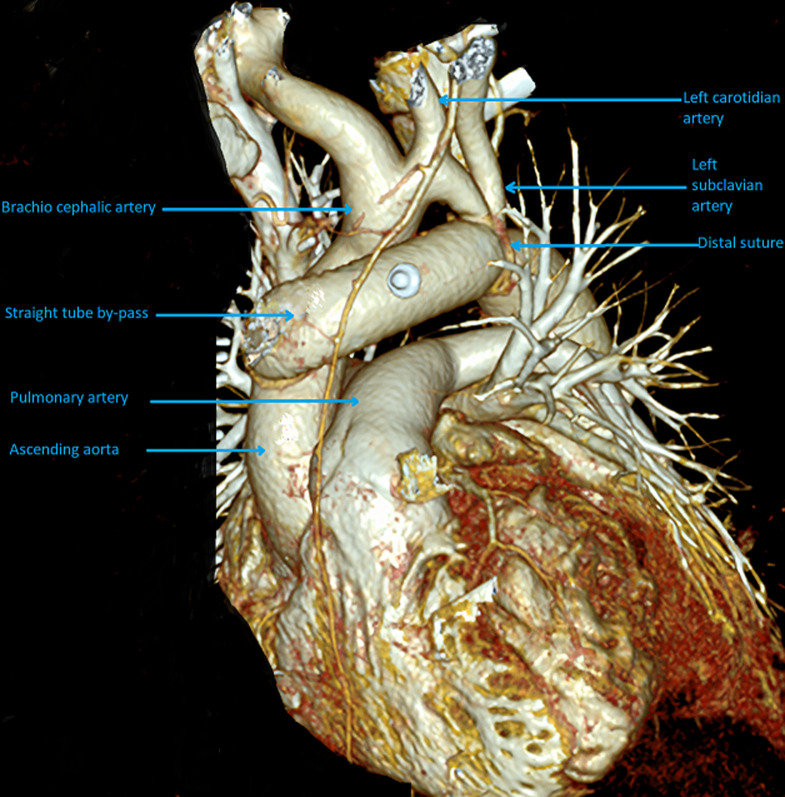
post-operative computed tomography angiography (CTA) reconstruction
